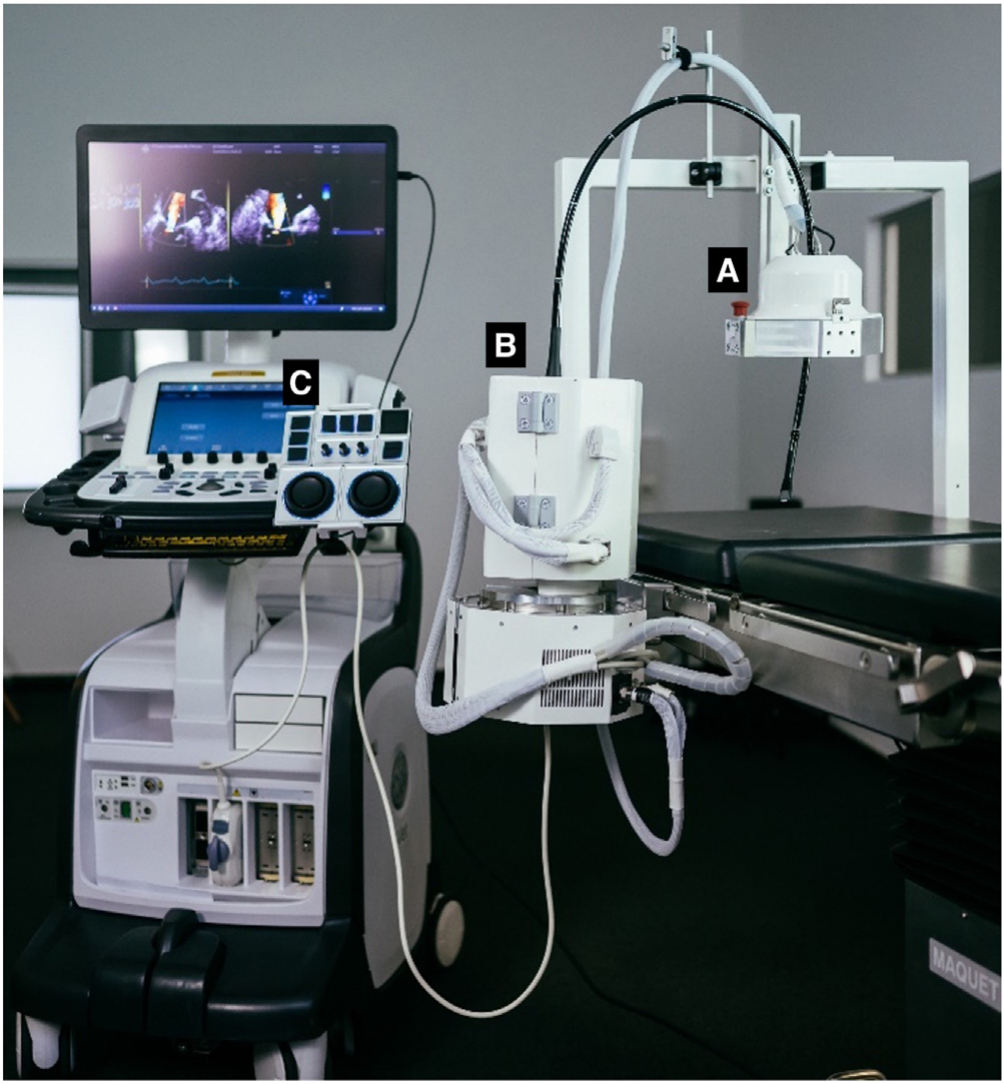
Conceptual design and setup of the ROB’E RCR system for TEE consisting of three parts: (a) ROB’E Guide; (b) ROB’E Base with the integrated ROB’E Computer; and (c) ROB’E Controller Abbreviations: RCR, remote-control robotic; TEE, transesophageal echocardiography.
